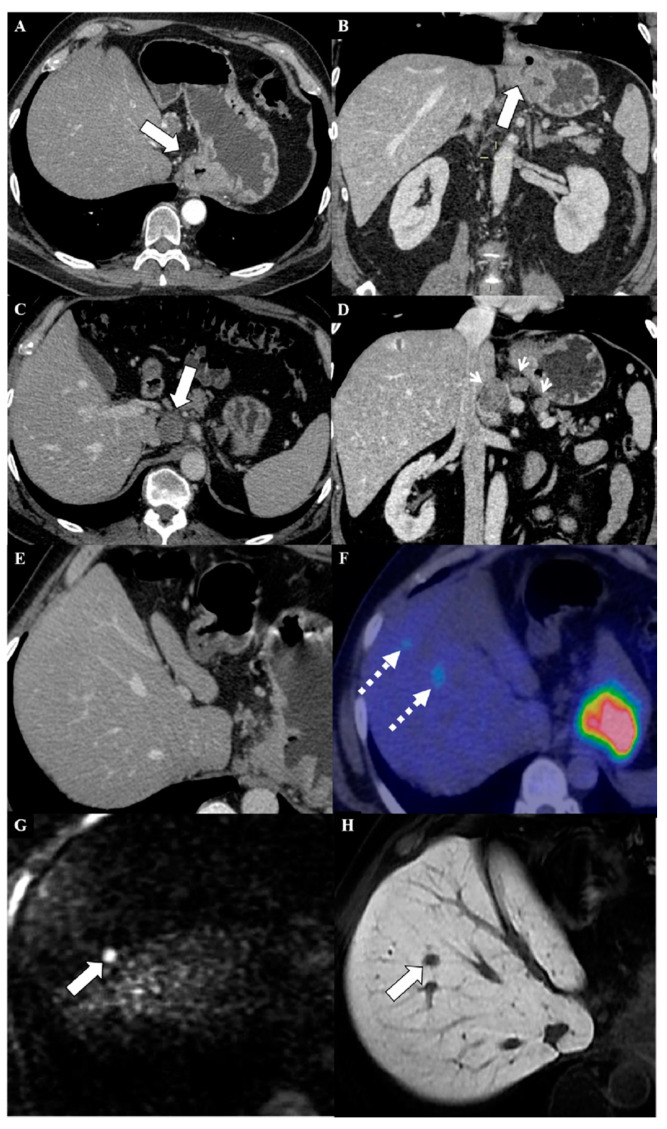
A 62-year-old man complaining of dysphagia and epigastric pain with advanced stage gastric cancer. Axial (A) and coronal reformatted (B) contrast-enhanced computed tomography (CT) images showed a circumferential soft-tissue thickening involving the gastroesophageal junction and the cardia of the stomach (white arrows) invading the serosa layer (T-stage: T4a). Multiple (3) enlarged lymph nodes were appreciable in the aortocaval (C) (white arrow) and celiac (D) (arrowheads) region (N-stage: N2). Axial contrast-enhanced CT scan (E) did not demonstrate liver metastasis, whereas the axial ^18^FDG positron emission tomography computed tomography (PET-CT) image (F) revealed two metabolically active lesions with the same uptake value (SUVmax 4.7) located in the VIII hepatic segment (dotted arrows), consistent with M1 disease. The subsequent axial gadoxetic acid-enhanced magnetic resonance imaging, performed using diffusion-weighted imaging (G) and during the hepatobiliary phase (H), confirmed only one metastatic lesion (white arrow).
